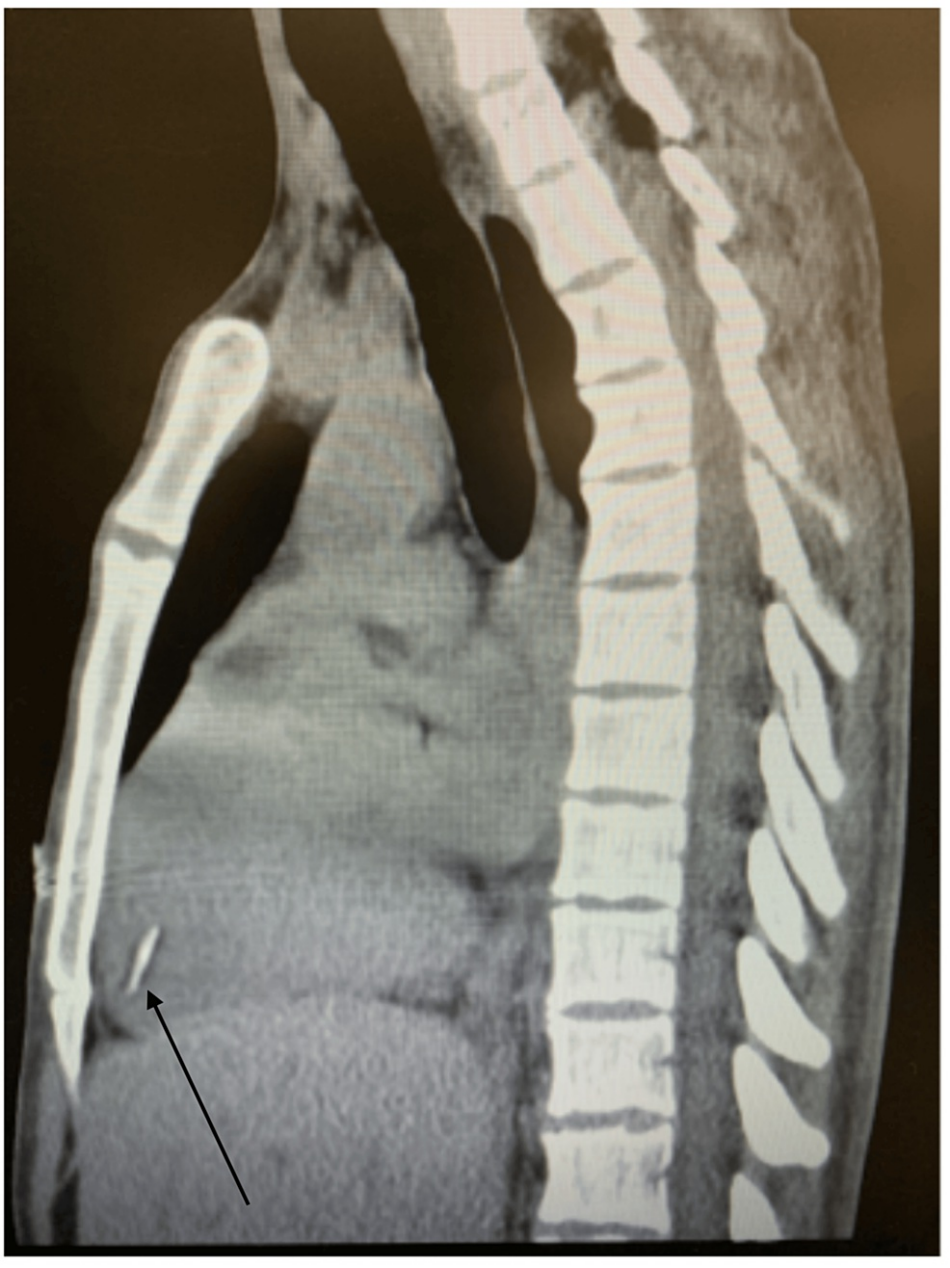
Chest CT sagittal view showing a radio-opaque foreign object in the right ventricle (black arrow).
